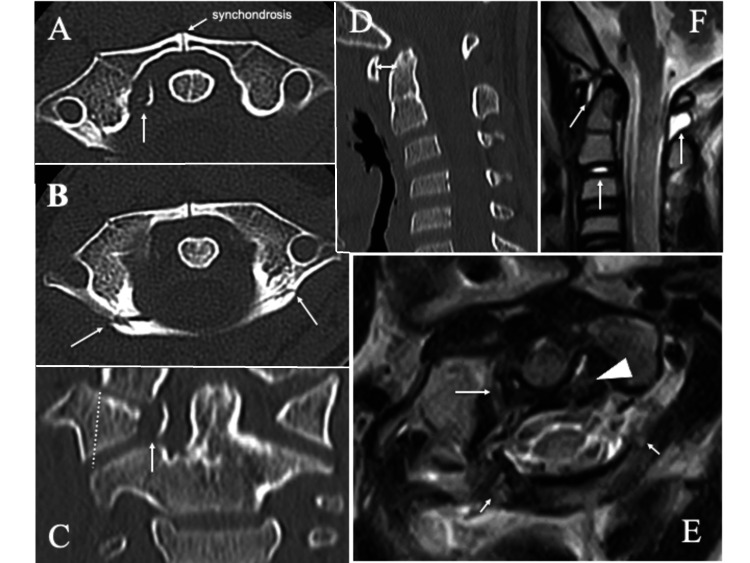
CT and MRI findings at initial presentation CT at initial presentation showing (A, C) an avulsion fracture of the right transverse atlantal ligament (arrows), (B) bilateral posterior arch fractures of the atlas (arrows), (C) slight lateral displacement of the right C1 lateral mass (dotted line), and (D) slight enlargement of the ADI (double-headed arrow). T2-weighted MRI showing (E) high signal intensity in the avulsed osseous tubercle of the right C1 lateral mass (arrow) and the fractured posterior atlantal arch (short arrows) and mild hyperintensity in the left substance of the transverse atlantal ligament (arrowhead) and (F) edematous or hemorrhagic changes around the odontoid process and in the cervical disc at C2/3 and in the posterior ligamentous complex at C1/2 (arrows). ADI: atlantodental interval
